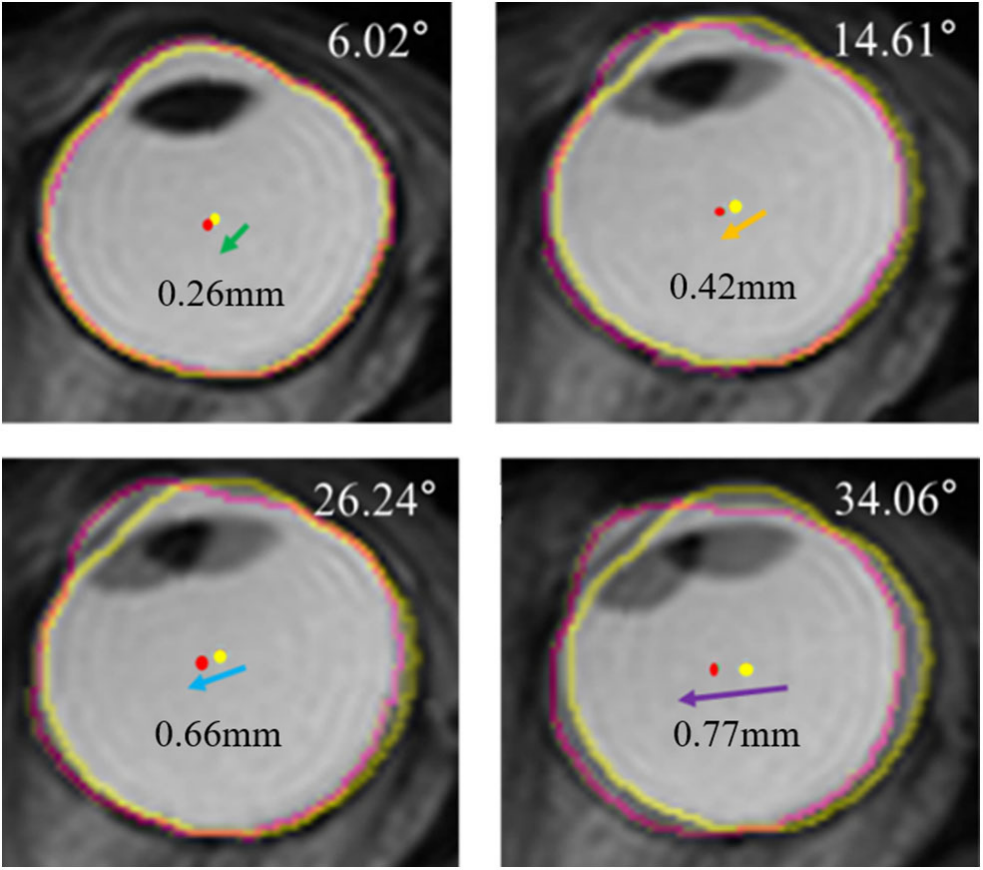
Serial superimposed MR images of right eye in a gradual increase of eyeball rotation. In a pilot study, one subject's MRI were obtained by increasing the angle of adduction gradually. After the identical processing, the extracted outline of the eyeball image is marked with a yellow line in central gaze and a pink line in left gaze. The centroid of the eyeball is marked with a yellow dot in central gaze and a pink dot in left gaze. Superimposed images show translations of eyeball, and the distance of translation increases when the rotation angle increases.
